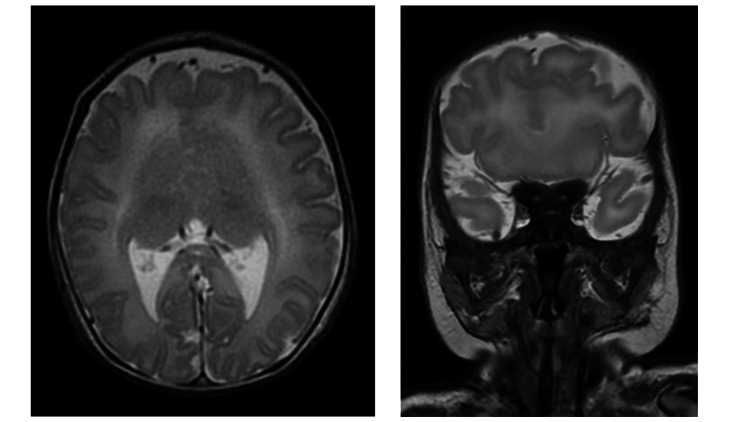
Brain MRI Axial (left) and coronal (right) images show dysplasia of the anterior commissure and fusion of the bilateral frontal lobes, findings consistent with semilobar holoprosencephaly. These findings indicate abnormalities in brain midline development, which could affect the hypothalamic-pituitary axis and help explain the cause of CDI. CDI: Central diabetes insipidus
